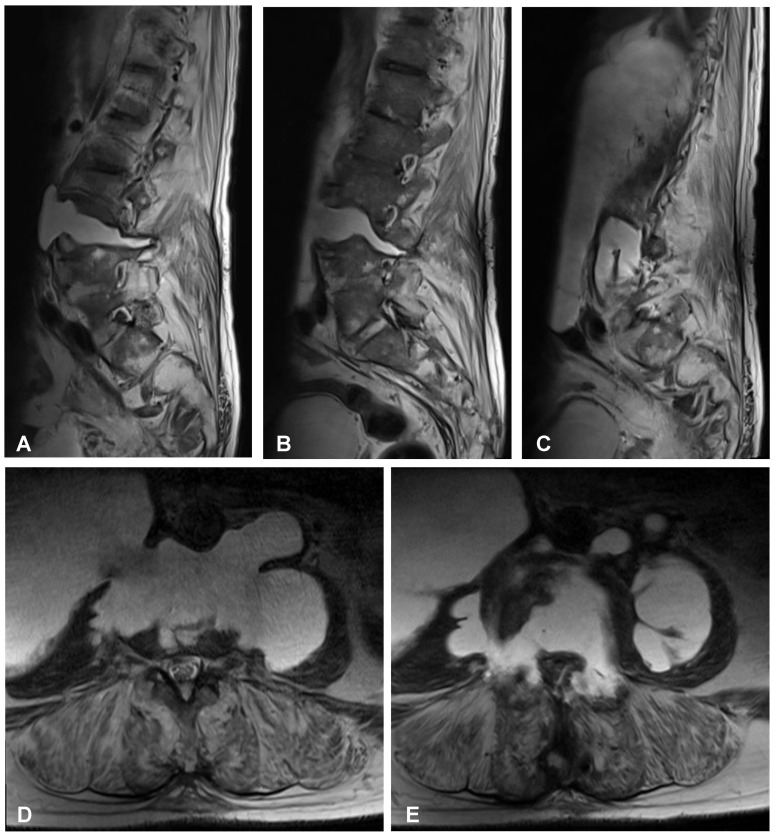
Preoperative sagittal (A–C) and axial (D,E) MRI scans (T2-weighted images) of the lumbar spine demonstrating high-intensity area at the L3/L4 intervertebral disc space communicating with both psoas major muscles forming massive well-defined fluid collection in the right retroperitoneum.
